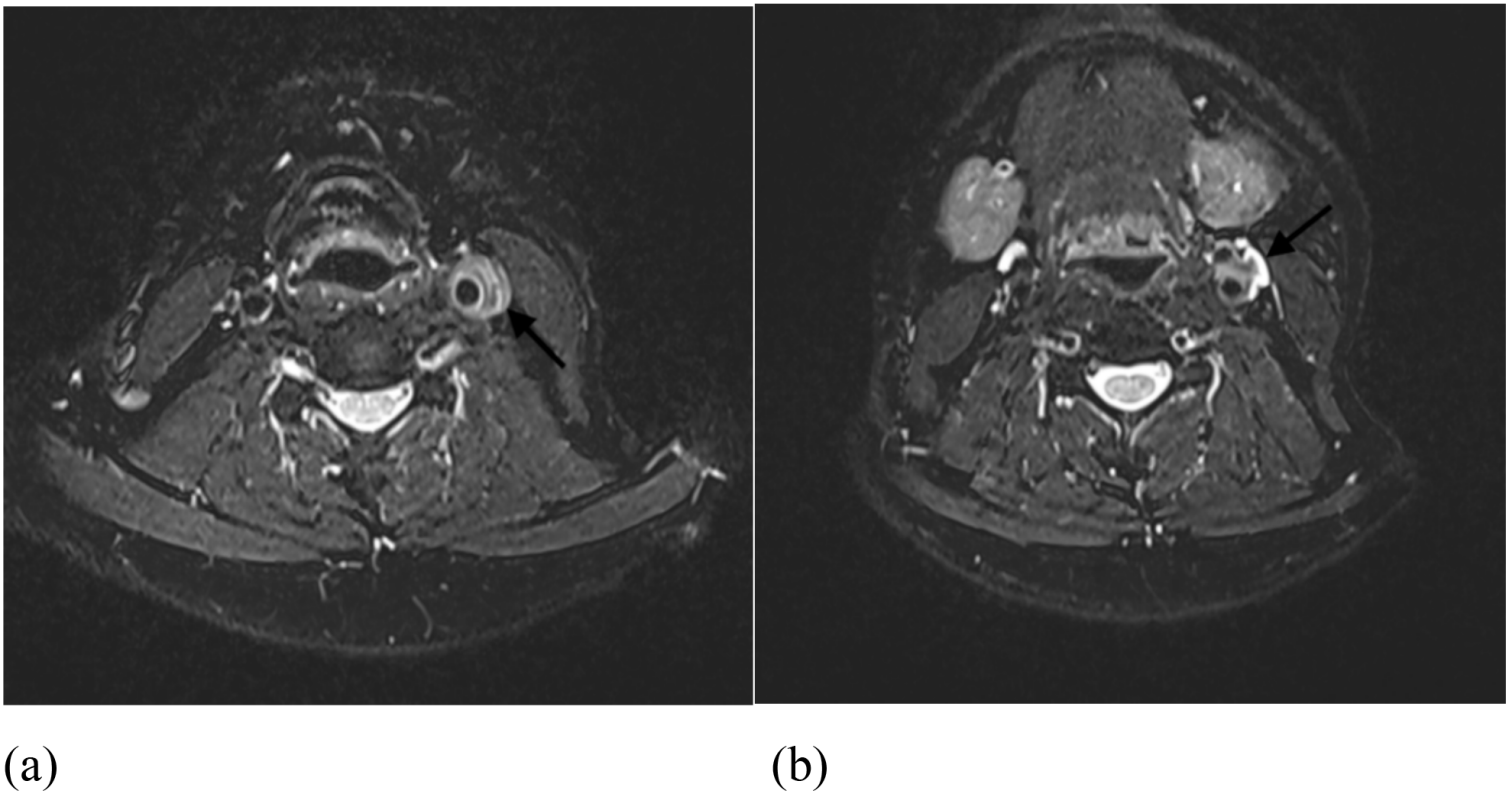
Contrast-enhanced neck MRI demonstrates: (a) Pericarotid mass lesions on T2-weighted sequences; (b) Mass lesions surrounding the carotid sinus on T2-weighted sequences.
